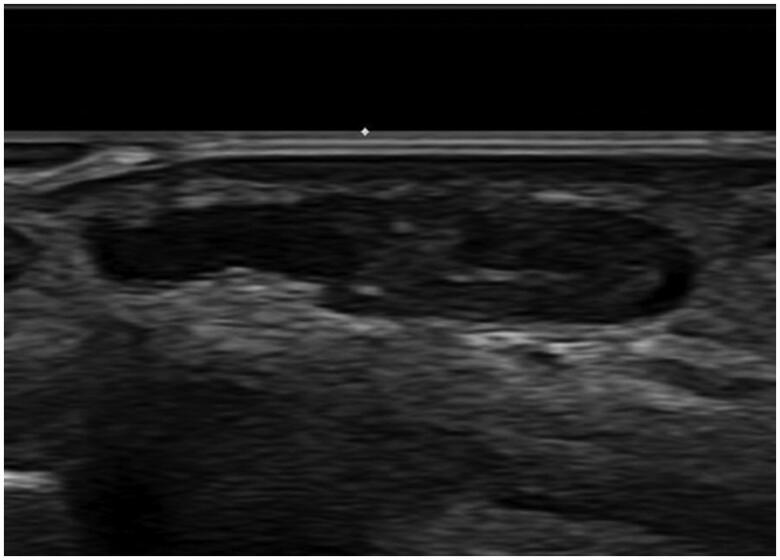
Preoperative ultrasonography reveals about 0.7 × 0.3 × 1.2 cm sized cystic lesion with no remarkable increased vascularity.
